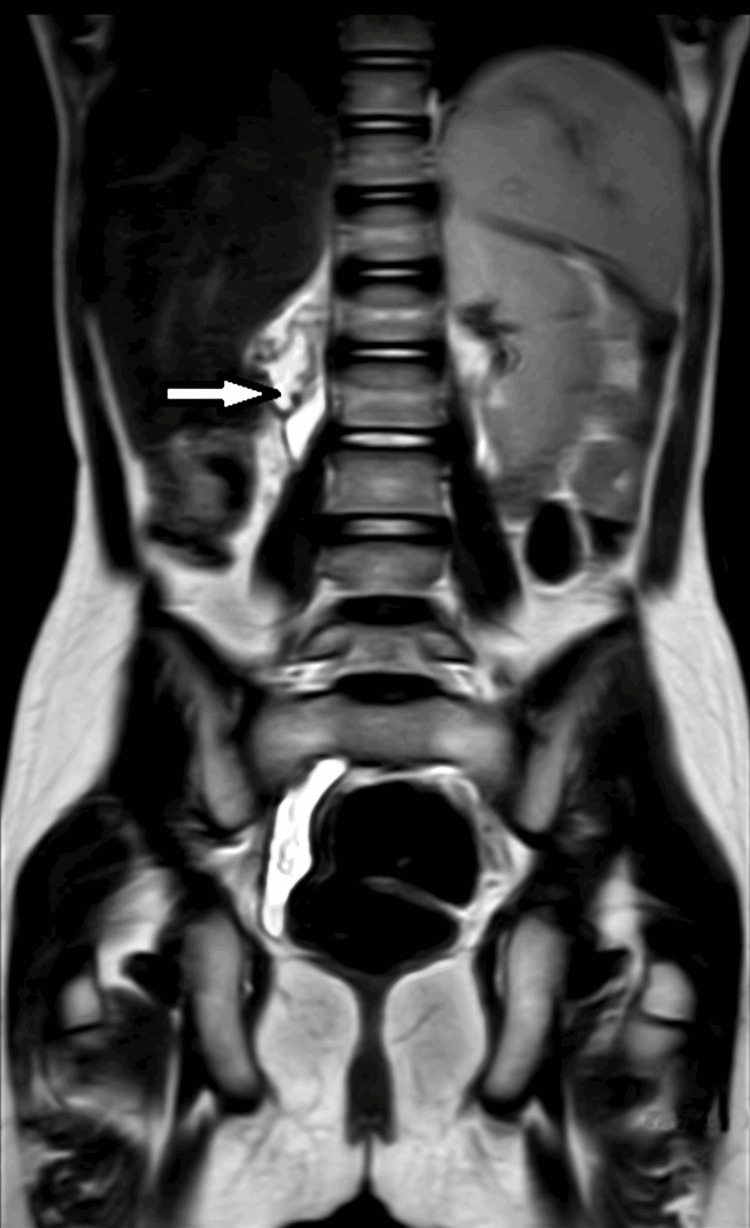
MRI showing atrophied right kidney (2.8 × 1 cm)
